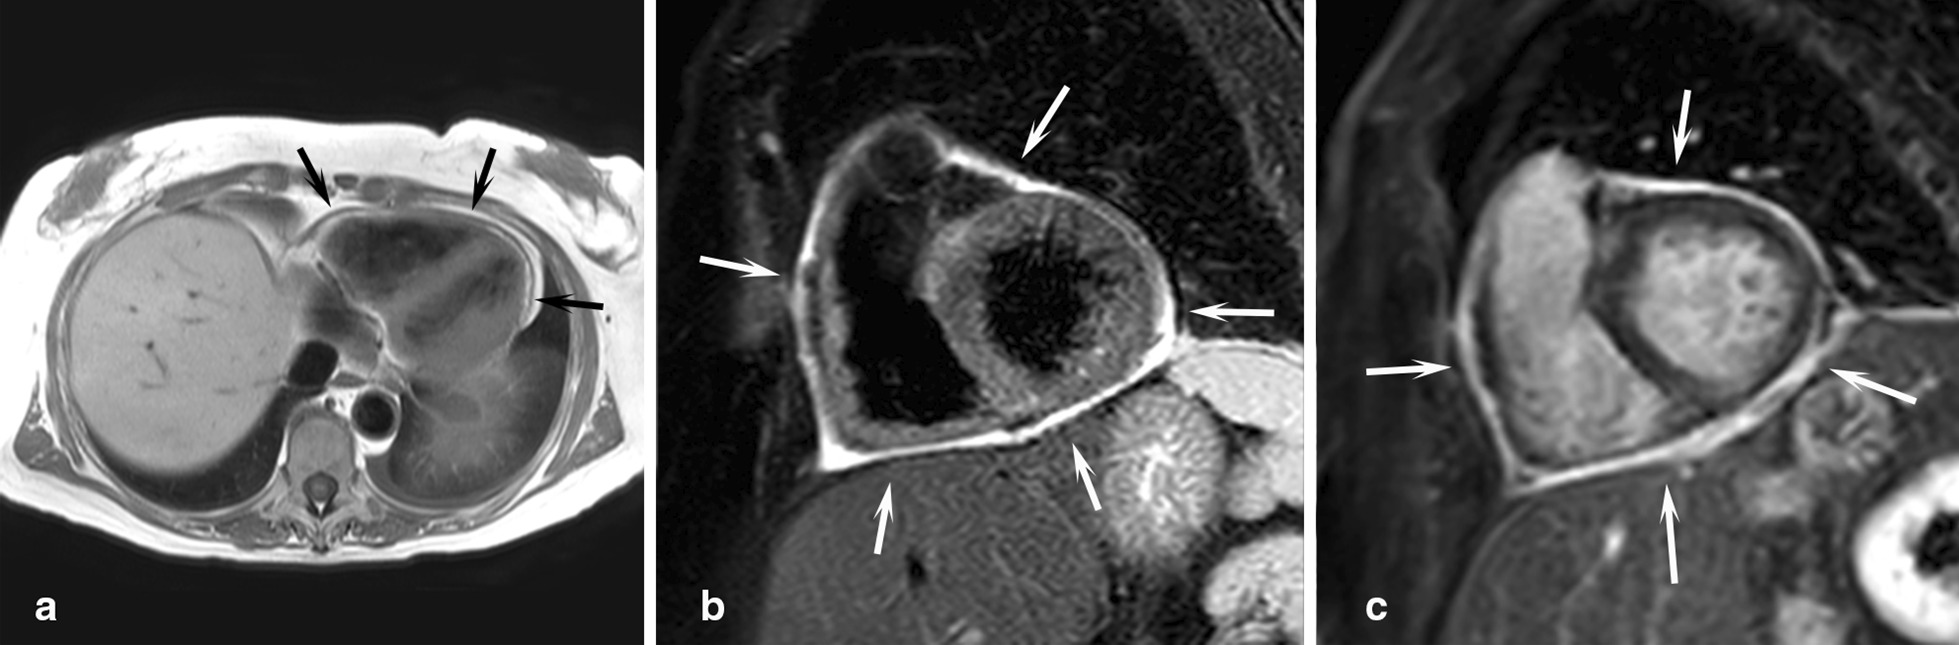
62-year-old woman with clinical presentation of Dressler’s syndrome following mitral and tricuspid valve replacement. Axial T1-weighted FSE imaging shows mildly thickening pericardium (a, black arrows). Short-axis T2-weighted fast spin echo imaging shows diffuse hyperintense appearance of the pericardium (b, white arrows). Late gadolinium enhancement imaging in cardiac short-axis shows diffuse strong pericardial enhancement (c, white arrows). CMR findings are highly suggestive of inflammatory pericarditis without evidence of associated pericardial effusion (“dry pericarditis”)
